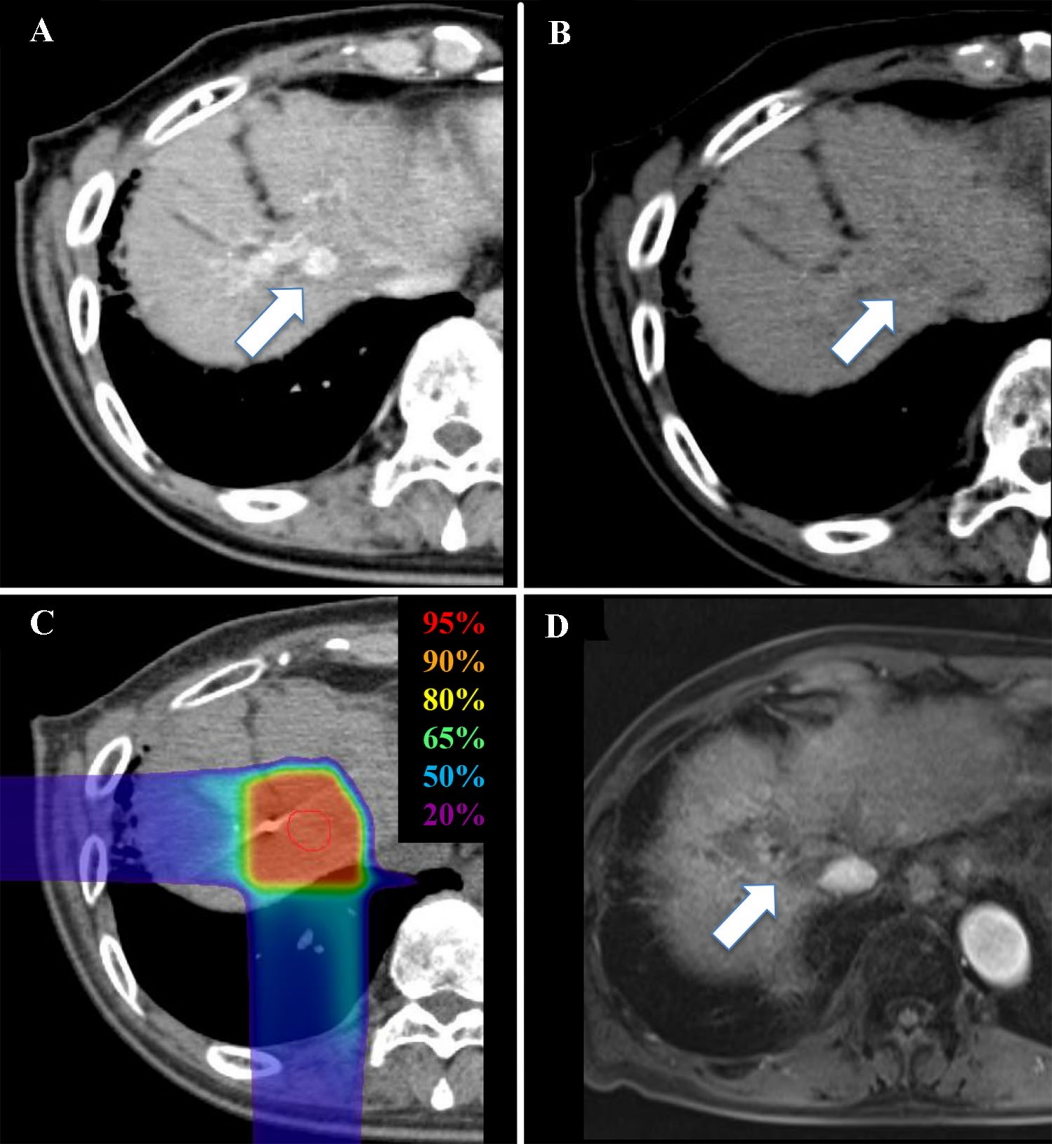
Computed tomography (CT), magnetic resonance imaging (MRI), and dose distribution of carbon-ion radiotherapy (C-ion RT) for local recurrence of hepatocellular carcinoma (HCC). a Axial CT image taken in the arterial phase. The white arrow shows recurrence of HCC with arterial enhancement. b CT image taken in the delayed phase. The white arrow shows slight washout. c Dose distribution of C-ion RT for recurrent HCC. The area within the red outline is the GTV. Highlighted are the 95% (red), 90% (orange), 80% (yellow), 65% (green), 50% (blue), and 20% (purple) isodose curves (100% = 60.0 Gy [RBE]). d MRI image taken in the arterial phase three months after re-irradiation with C-ion RT. The white arrow shows tumor shrinkage
